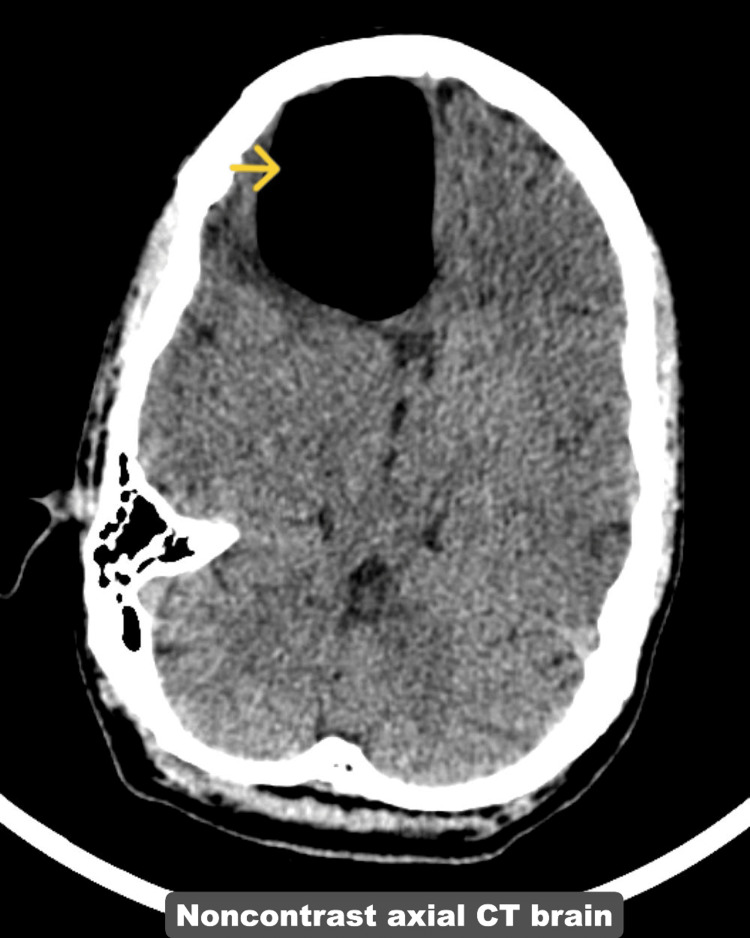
Day 40 non-contrast axial CT brain showing large intracerebral pneumatocele with mass effect Yellow arrow shows large intracerebral pneumatocele in the right basifrontal region.
